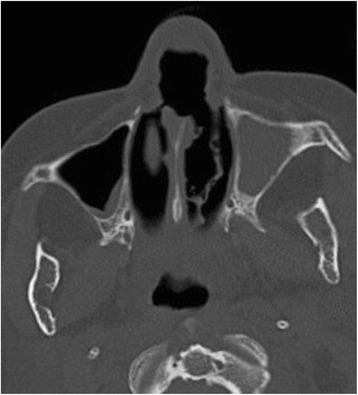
Mucosal leishmaniasis. CT scan showing left maxillary sinusitis and centimetric perforation of the nasal septal cartilage
